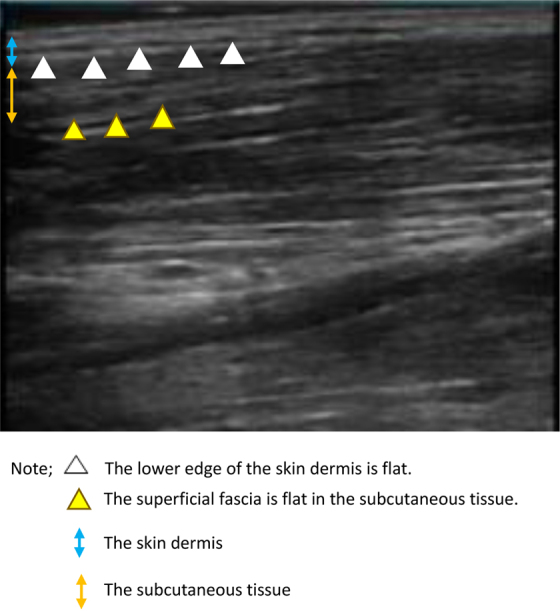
Findings in the lower limbs of patients who did not develop edema of ultrasonic images of 15 cm below the fibula head.
